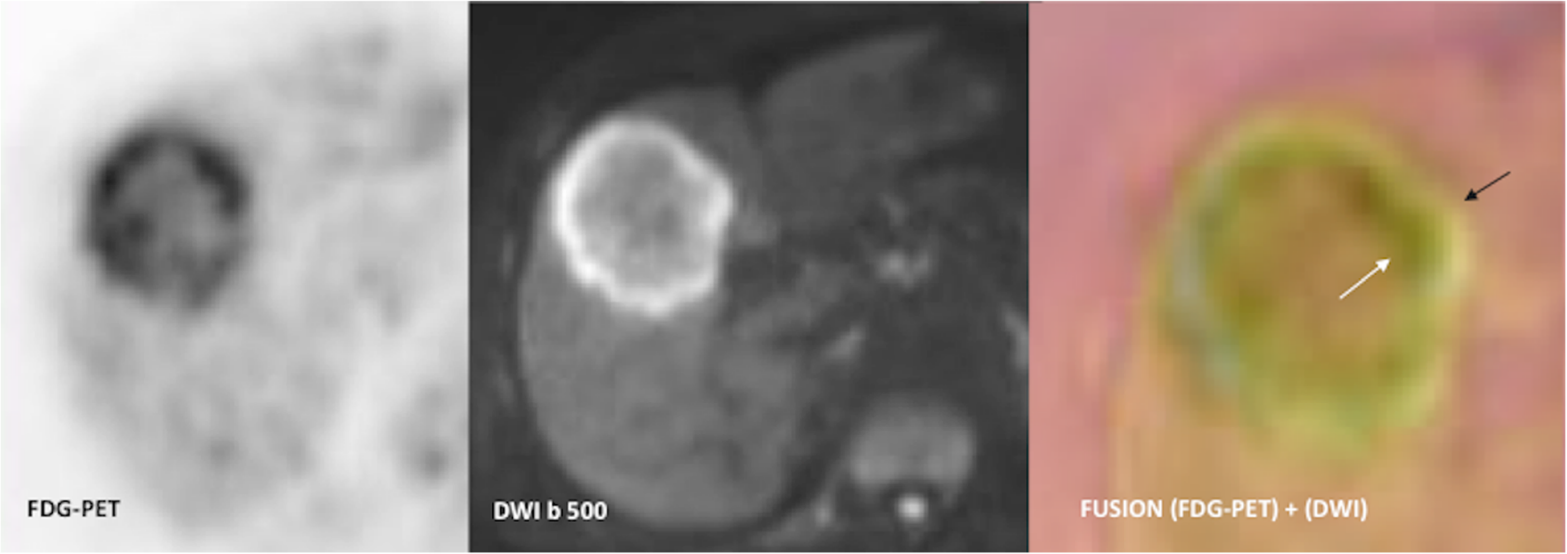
Colorectal cancer liver metastasis in a 56-year-old man. FDG-PET (left) and b500 diffusion-weighted (middle) images demonstrated the presence of a mismatch between the obtained parameters. Note that the size of the FDG abnormality is smaller than the diffusion one (black arrow) (right). Tumor biology may explain this feature. Higher FDG uptake occurs at the edge of the necrotic cavity (white arrow) which is of relative low SI on b500 image. The edge of the necrotic cavity usually represents an area of relative tumor hypoxia, which may promote a high metabolic activity (vascular metabolic-mismatch). On its part, the periphery of the mass generally presents good perfusion and it is the most cellular area of the tumor, explaining the restricted diffusion at this level
